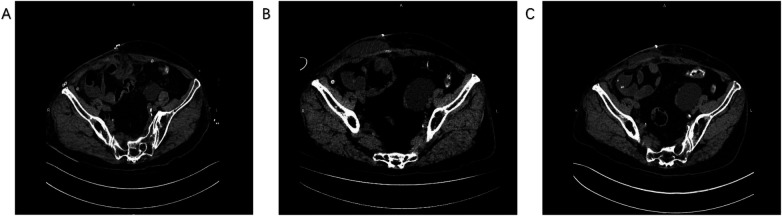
A is the abdominal CT scan on the third day after surgery, B is the abdominal CT scan on the seventh day after surgery, and C is the abdominal CT scan on the fourteenth day after surgery.
